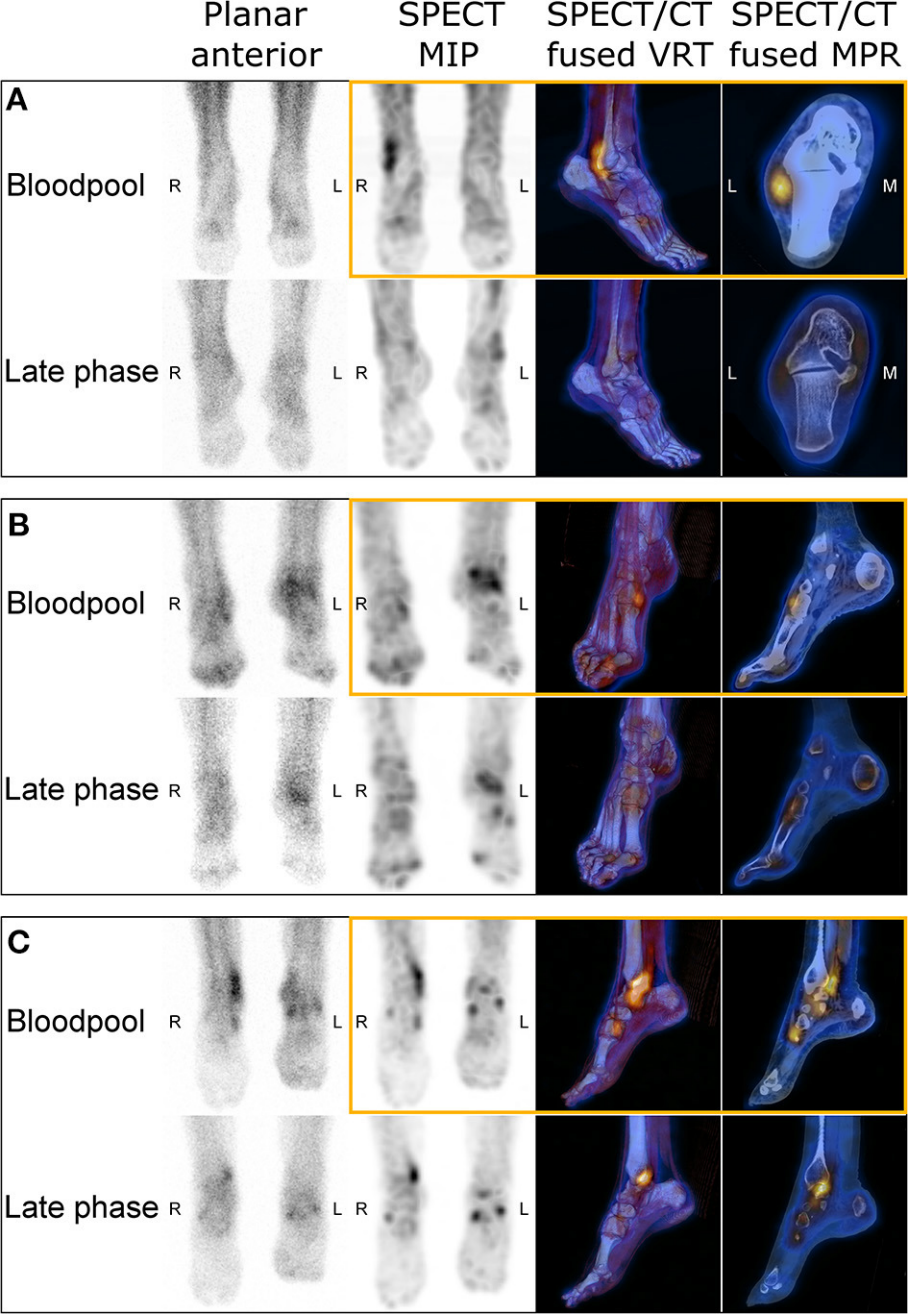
Images of three-phase bone scintigraphy from three patients with right lower extremity pain, including images provided by bloodpool SPECT/CT (in orange boxes). Patient (A) was a 62-year-old man referred for suspicion of right lateral malleolus stress fracture. Bloodpool SPECT/CT revealed intense uptake along peroneal tendons, highly suggesting the diagnosis of right peroneal tendonitis. Patient (B) was an 81-year-old woman with pain of the right ankle and anterior tarsus, suspected with stress fracture. Bloodpool SPECT/CT revealed focal uptake at the insertion of the anterior tibial tendon, leading to set the diagnosis of right tibial anterior tendonitis. Patient (C) was a 60-year-old woman, suffering from right medial ankle pain. Planar bloodpool and late phase SPECT/CT images were compatible with a posterior tibial tendonitis with a low diagnostic confidence. Bloodpool SPECT/CT substantially increased the diagnostic confidence for posterior tibial tendonitis and revealed intense focal uptake at the insertion of the anterior tibial tendon, evocative of associated right tibial anterior tendonitis.
